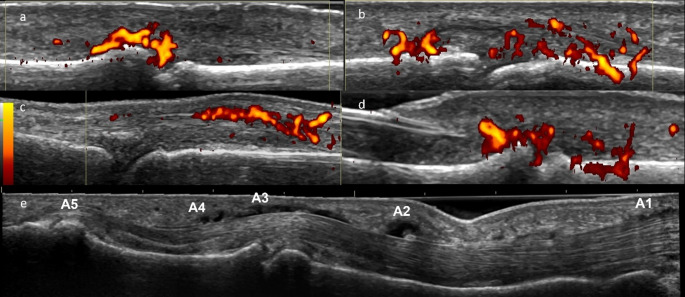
Small hand enthesitis in patients with PsA. Legend. (a) Active enthesitis of the finger extensor tendon central slip at its insertion into the middle phalanx acquired using a dorsal longitudinal scan at the proximal interphalangeal joint level; (b) Radial collateral ligament enthesitis of the index finger, visualized at its insertion on the proximal phalanx using a lateral longitudinal ultrasound scan. (c) Peritendinitis of the finger extensor tendon, acquired using a longitudinal dorsal scan at the metacarpophalangeal joint level; (d) Distal interphalangeal joint enthesitis of the extensor tendon insertion into the distal phalanx, imaged with a dorsal longitudinal scan at the distal interphalangeal joint level; (e) Dactylitis of the middle finger with inflammation of multiple flexor pulleys, and tendon sheath effusion, acquired using a volar longitudinal scan along the entire digit.
